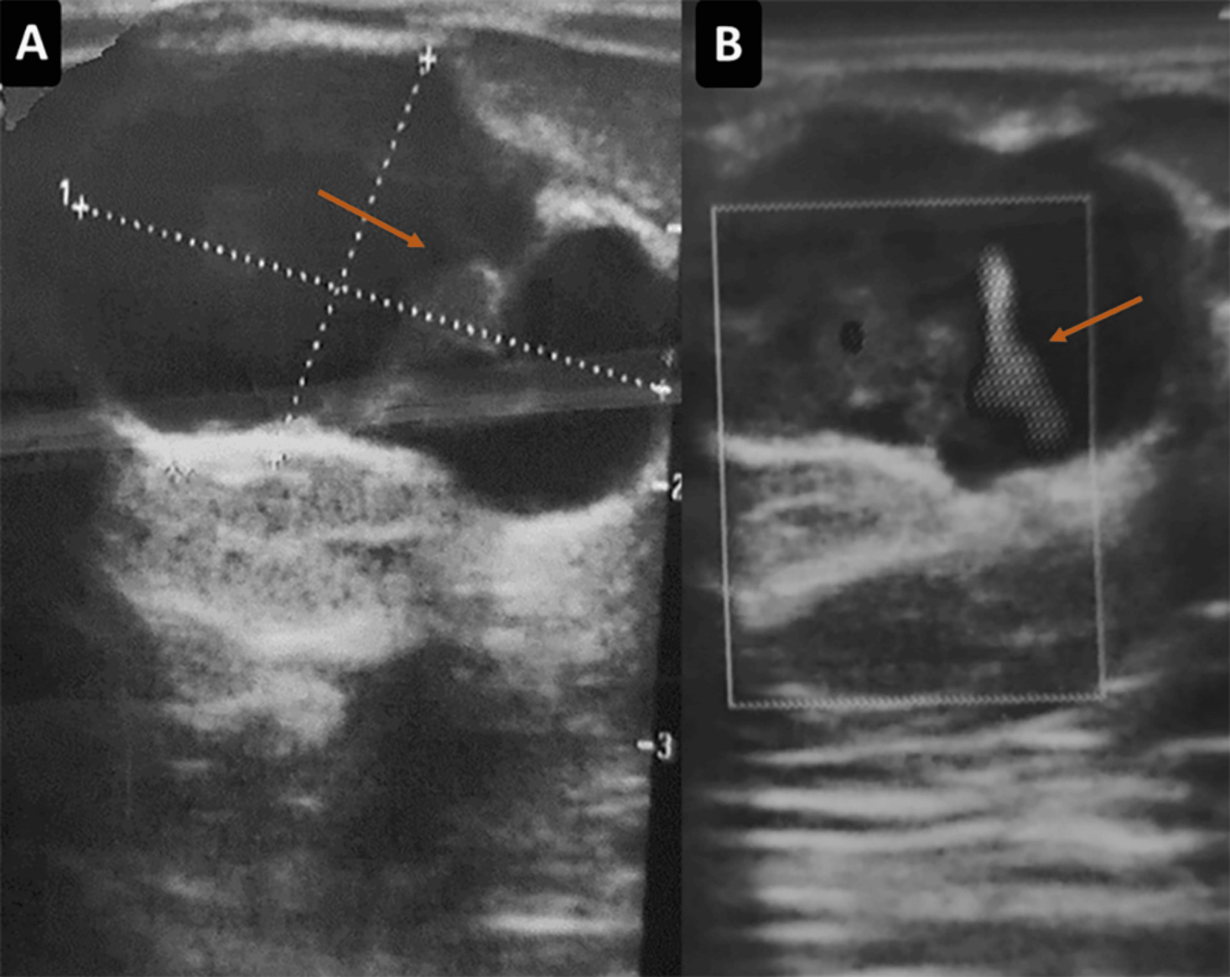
Breast ultrasound (first case). Oval, circumscribed, parallel, predominantly anechoic mass with a tissular component (arrow) (A), demonstrating vascularity on color Doppler (B), measuring 31 × 17 mm.
